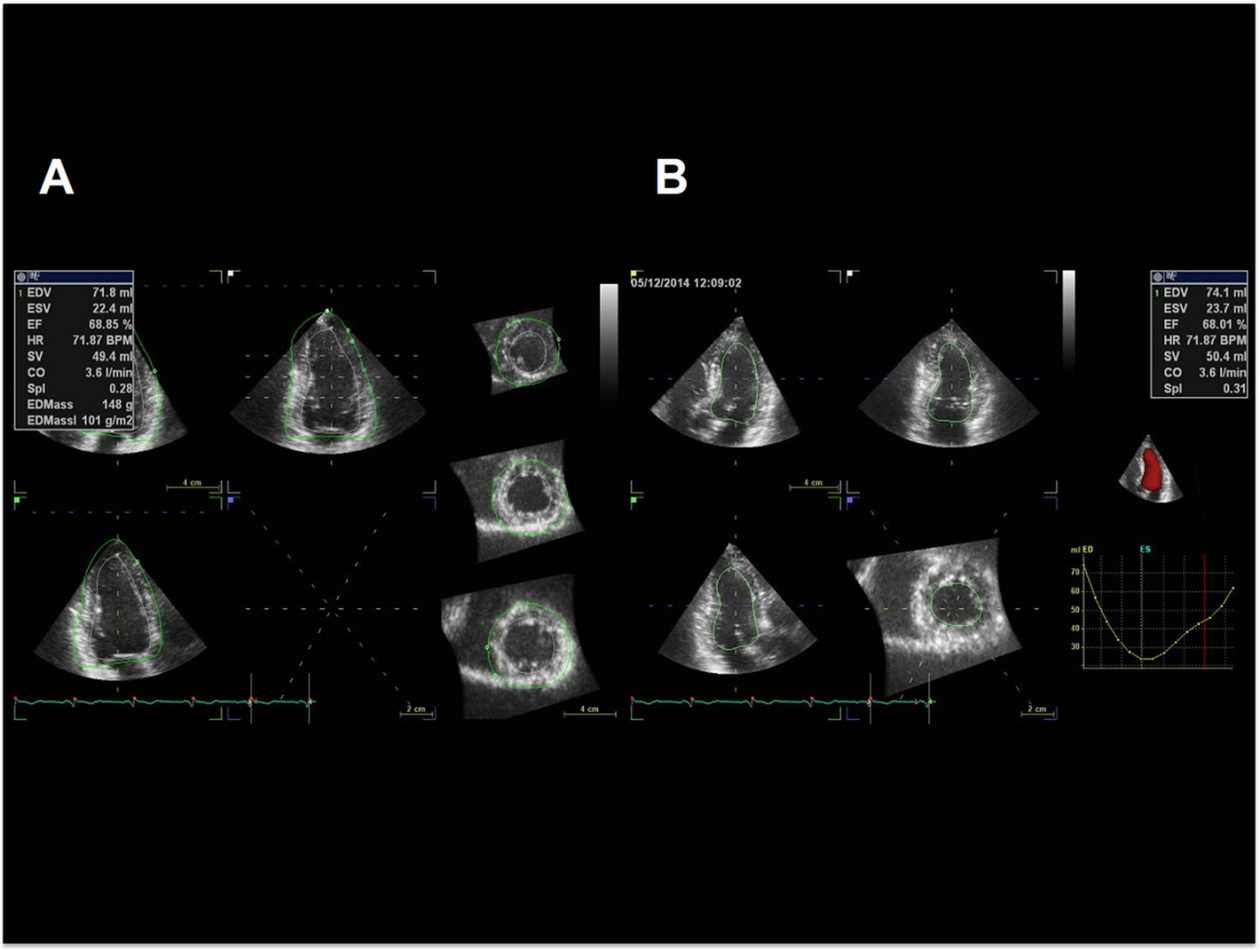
Examples of calculation of left ventricular mass (A) and volumes (B) by three-dimensional echocardiography.
